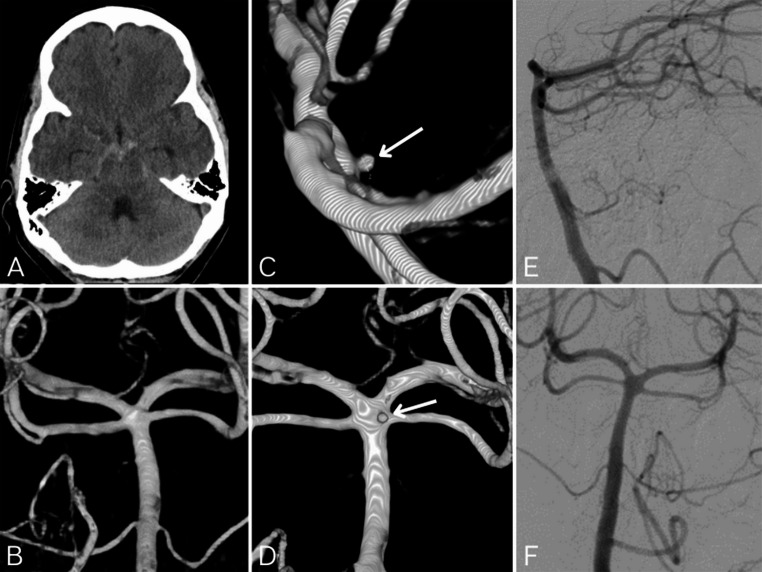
A. Non-contrast cranial CT showing evidence of a perimesencephalic subarachnoid hemorrhage B. First digital subtraction angiography (DSA) with 3D rotational angiography (frontal view) from the left vertebral artery, revealing no bleeding source such as an aneurysm C, D. Second DSA performed 10 days later with 3D rotational angiography from the left vertebral artery: (C = lateral view; D = frontal view). A small dorsally oriented aneurysm (0.8 × 1 mm) of the distal basilar artery is identified (arrows) E, F. Second DSA with standard angiographic series from the left vertebral artery: (E = lateral projection; F = frontal projection). Despite 3D rotational angiography, the small basilar artery aneurysm remains undetectable on standard projections
